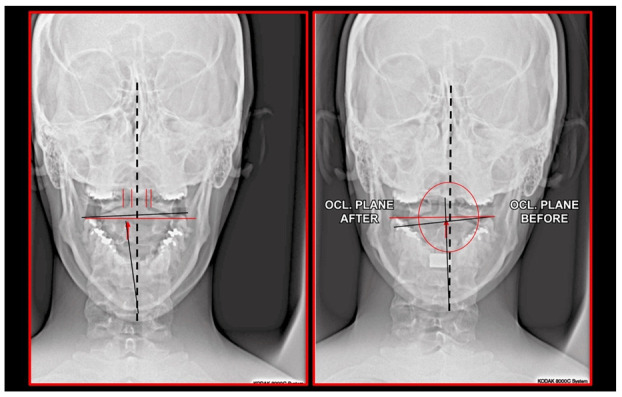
Radiographic situation before and after one session of orthopedic manual therapy.
